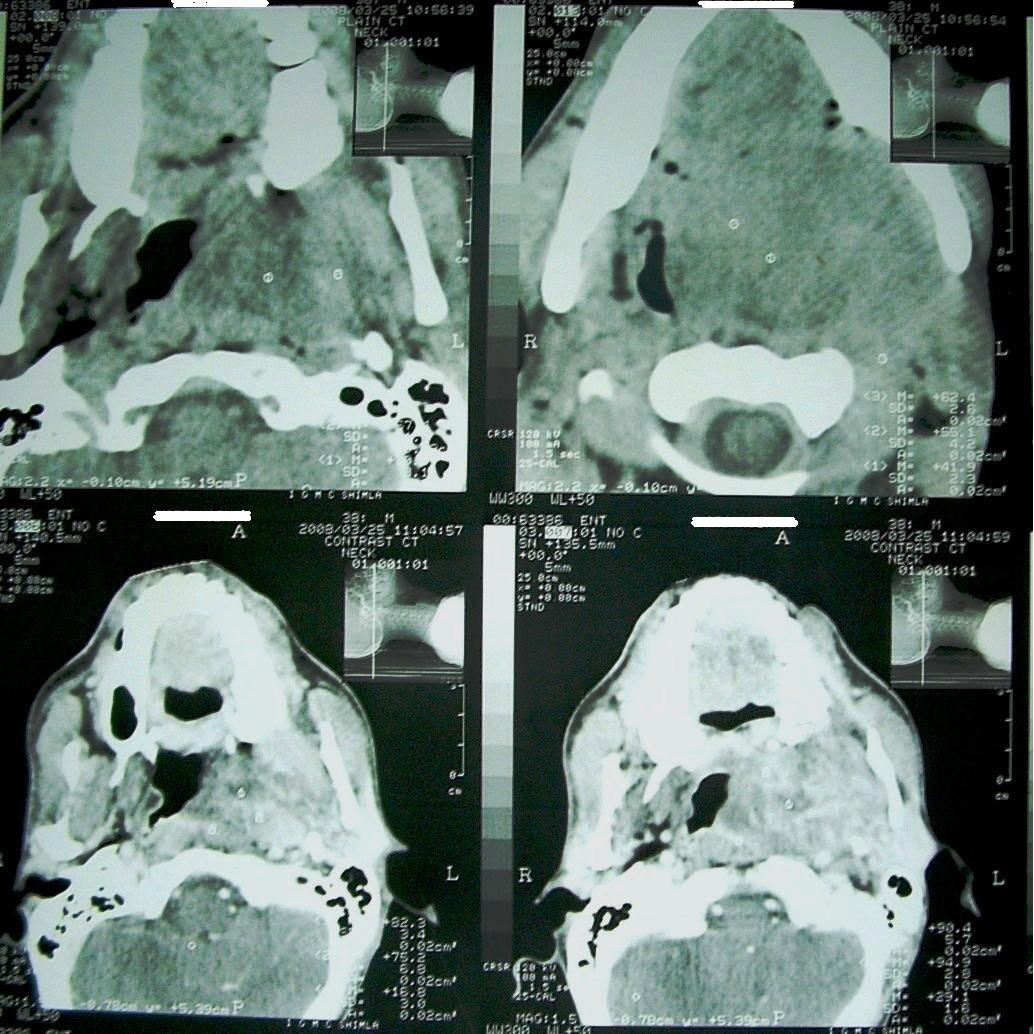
On 25^th ^March 2008, post-radiotherapy CT scan showed same heterogeneous enhanced tumor of nasopharynx but increased considerably as compared to earlier CT scan (Fig 1).
